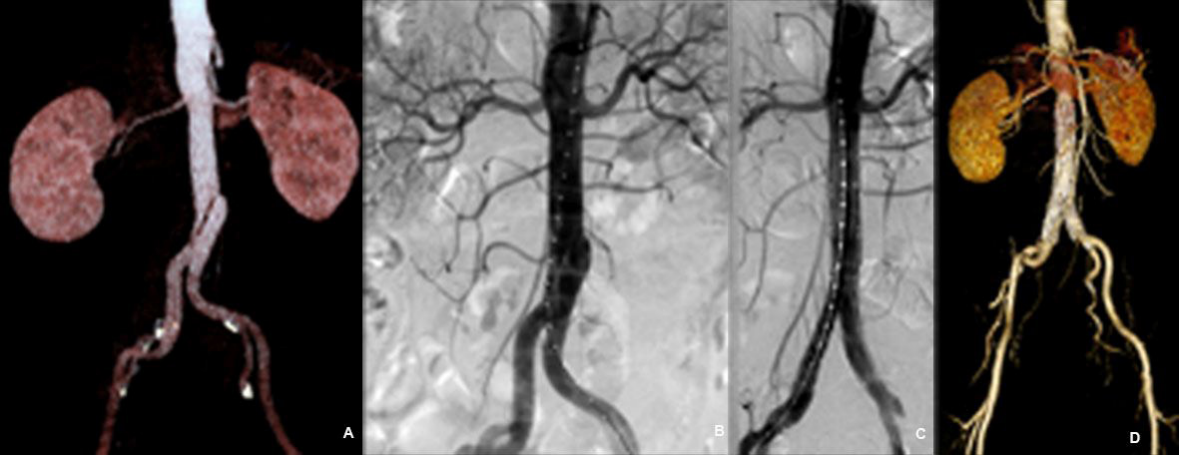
Example of isolated abdominal aortic dissection treated with main unibody bifurcated stent graft device only. (A) Pre-treatment CTA image; (B) Intraoperative angiography; (C) Post-treatment angiographic appearance; (D) Post-treatment control CTA.
